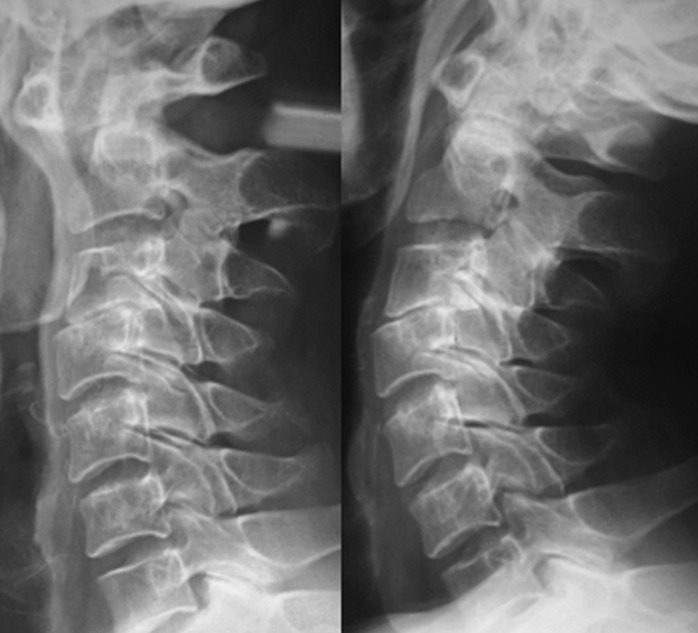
dynamic X-rays showing overt C1-C2 instability and C3/C4 retrolisthesis
